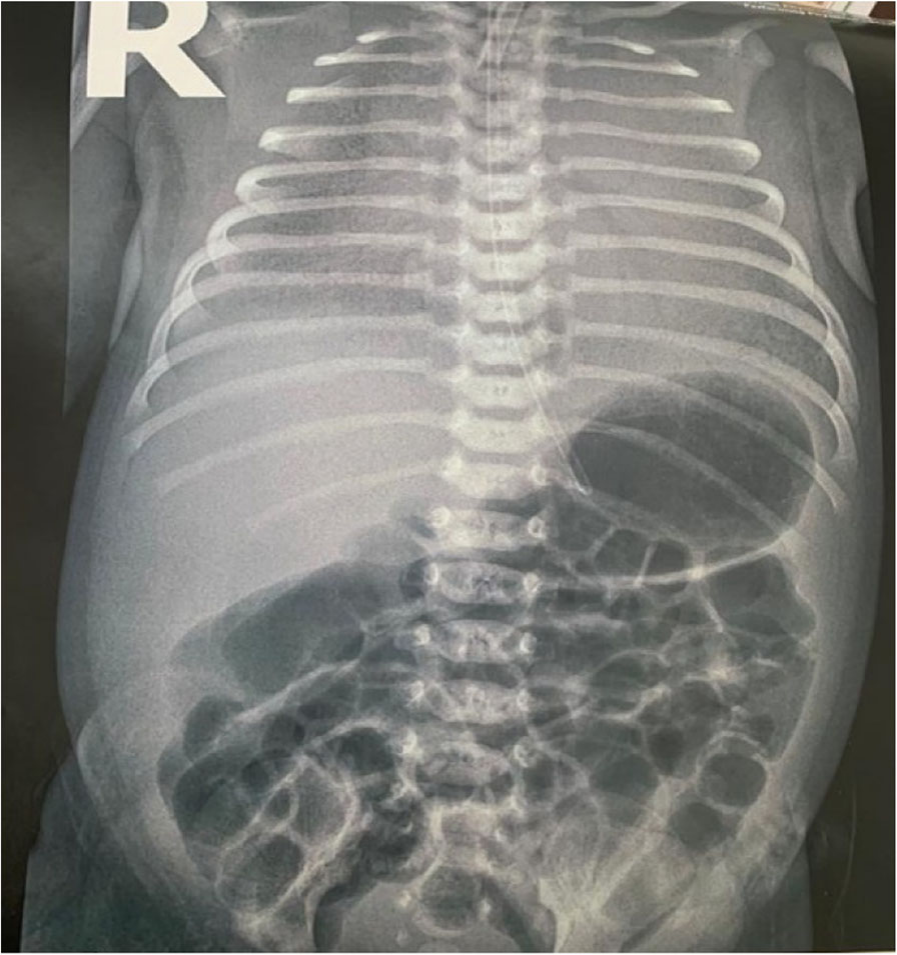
Newborn chest radiography
